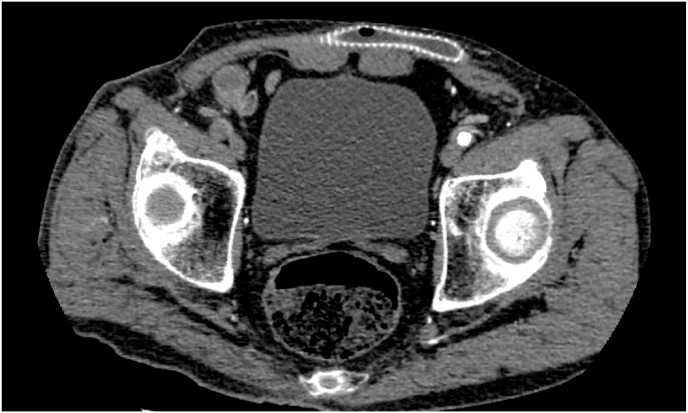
A) Computed tomography showing an infected abscess in contact with an air bubble in the graft. B) Computed tomography showing an air bubble in the femoral-femoral crossover bypass graft.
